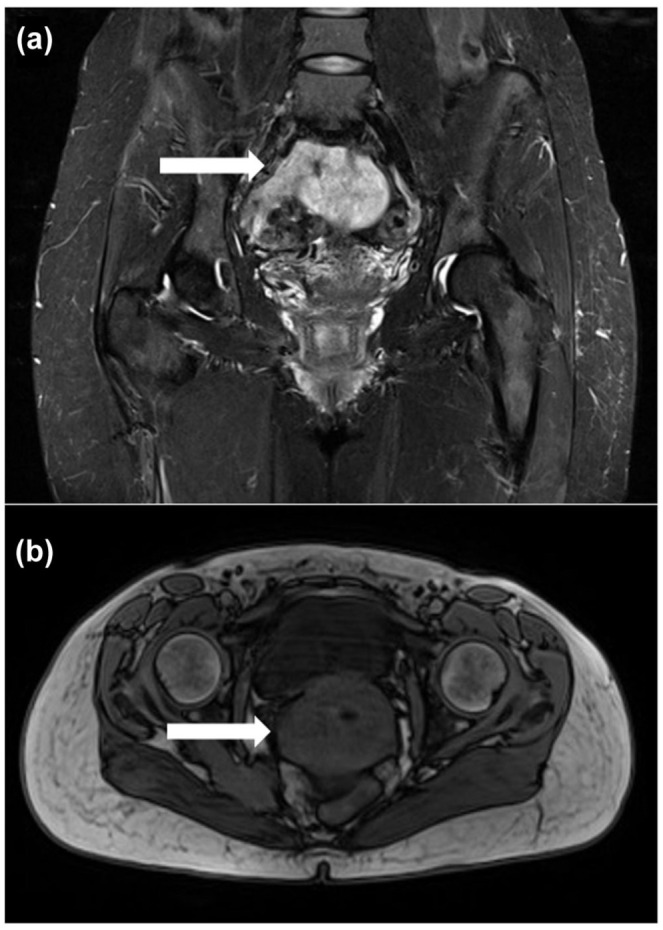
The MRI image of a pelvic mass of leiomyosarcoma on the right side: (a) contrast-enhanced coronal view and (b) axial view without contrast. MRI-magnetic resonance imaging.
